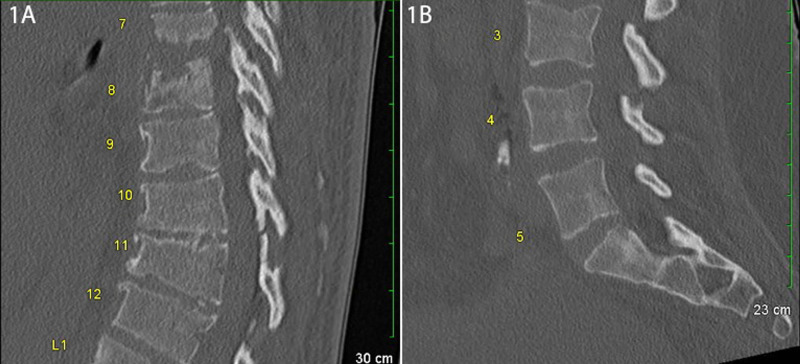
Sagittal CT of thoracic and lumbar spine. (A) Sagittal CT of the thoracic spine reveals a destructive process at T7-T8 and T10-T11 disc spaces and adjacent vertebral bodies suggestive of discitis and/or osteomyelitis. (B) Sagittal CT of the lumbar spine reveals no acute abnormalities. CT = computed tomography
